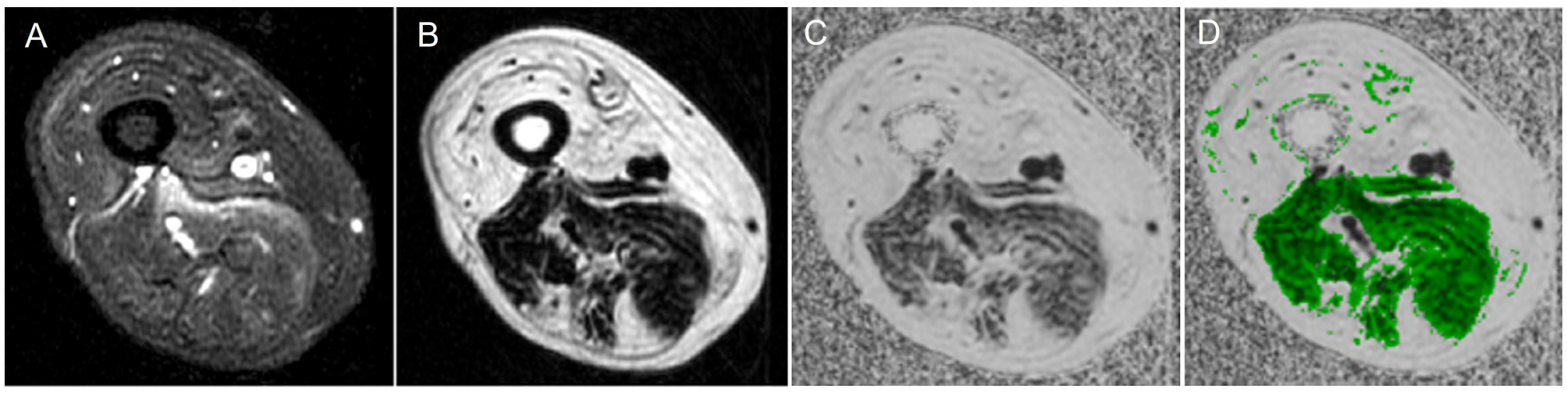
Magnetic resonance imaging of muscle showing water vs. fat image and a fat fraction map. (A) Water image, (B) fat image, (C) fat fraction map, and (D) fat fraction map with muscle region overlaid.
